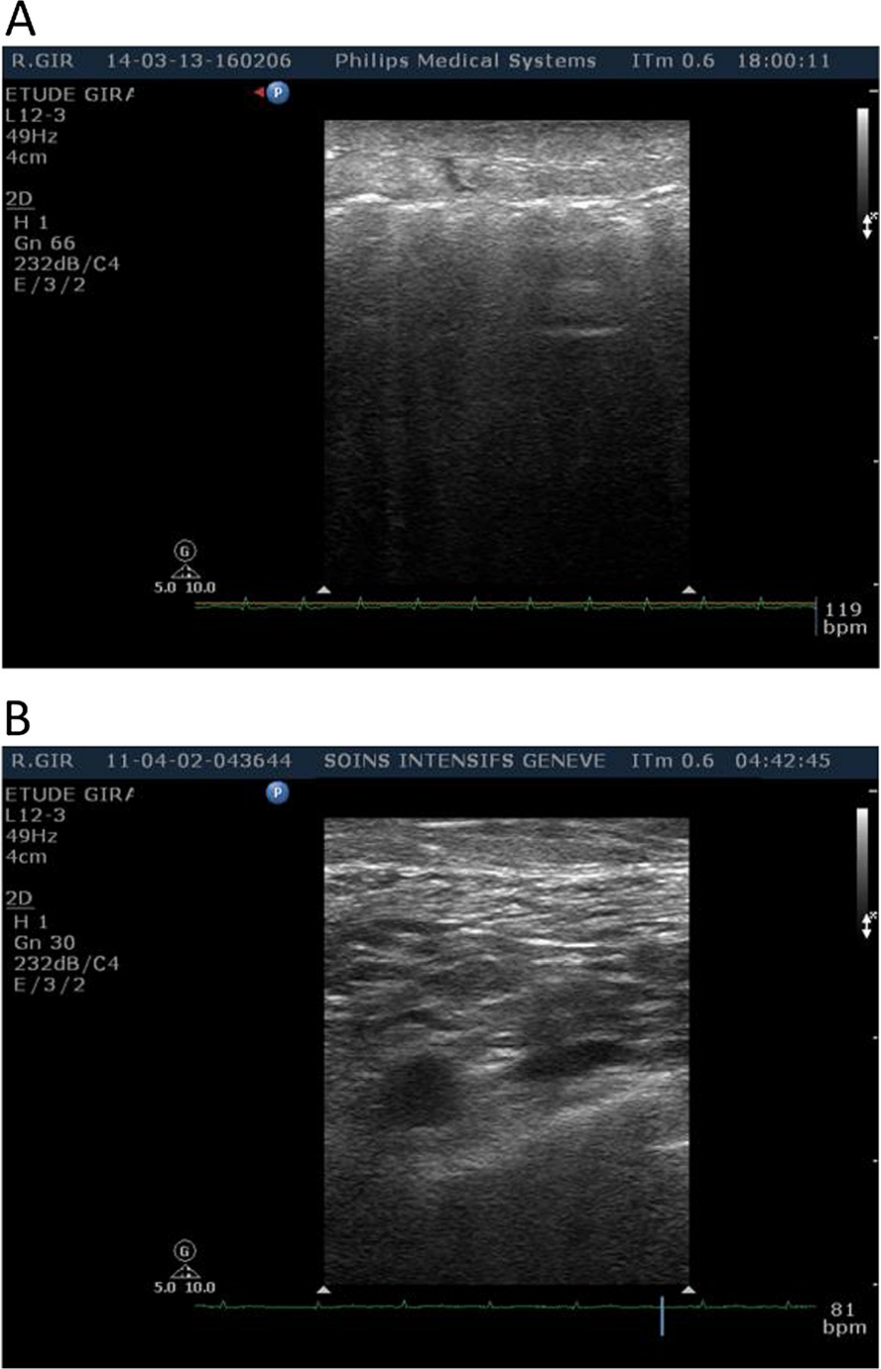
Ultrasound images of the neck for the internal jugular vein location during vascular access puncture (A) with and (B) without subcutaneous emphysema. An impossible ultrasound guidance (A) and a possible ultrasound guidance (B) are shown.
